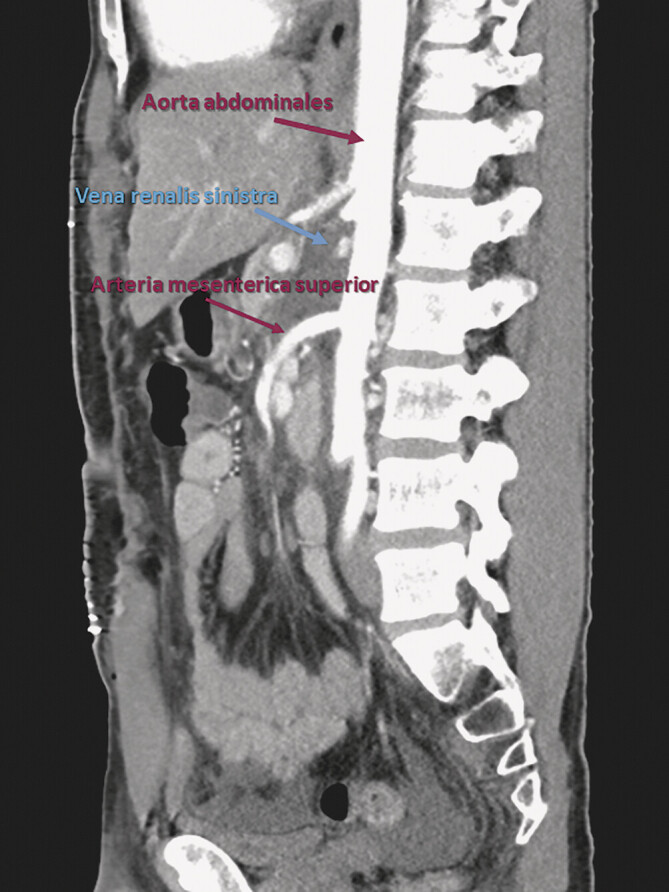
CT des Abdomens – sagittale Rekonstruktion mit arterieller Kontrastmittelphase: Nach distal auf die Aorta abdominalis transponierte A. mesenterica superior mit nun dekomprimierter V. renalis sinistra.
